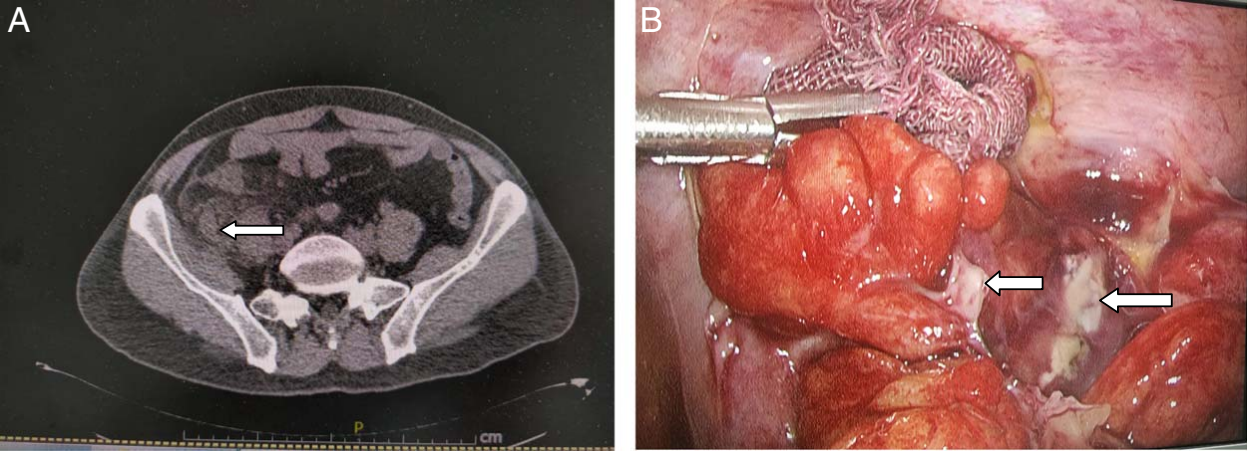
Imaging findings of CT scan and laparoscopy. The appendix was enlarged with peri-appendiceal fat stranding and fluid (arrow ⇦) around it (A); laparoscopy showed purulence (arrow ⇦) around the area of the appendix (B).
